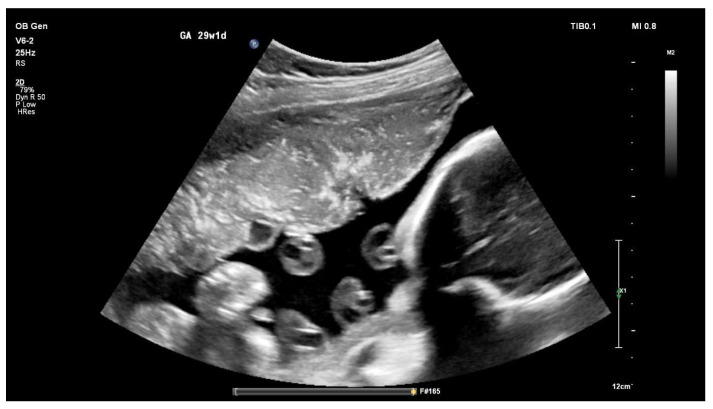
Obstetric ultrasound image showing a heterogeneous placenta with multiple echogenic foci consistent with placental calcifications (advanced placental maturation).
